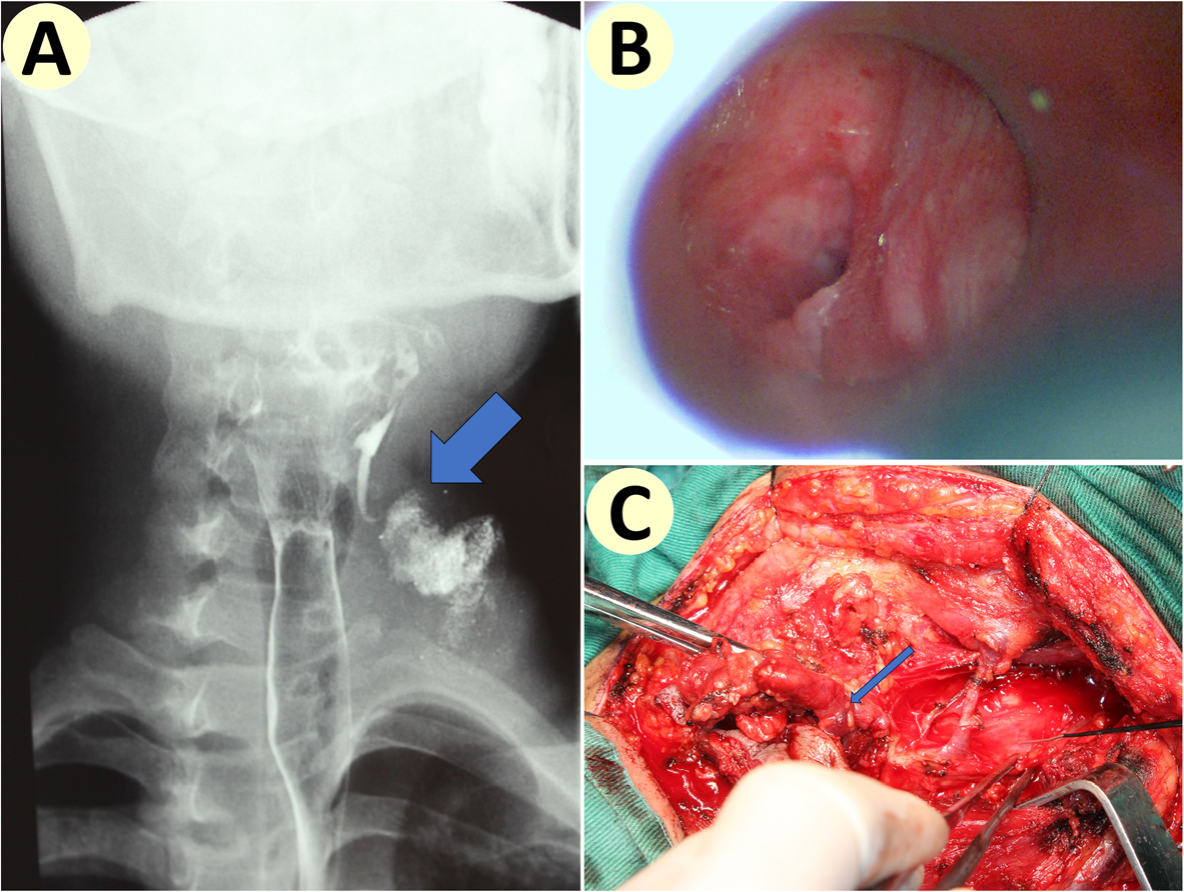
Pre-operative diagnosis and intra-operative confirmation. a Outflow of barium from pyriform sinus into the fistula tract and further into the massive cervical lesion could be seen on X-ray film (see blue arrow). b Intra-operative direct laryngoscope visualized the internal orifice at the apex of pyriform fossa of the same patient. c Intra-operative confirmation of entry into pyriform fossa at the inferior cornu of thyroid cartilage (see blue arrow) of the same patient
